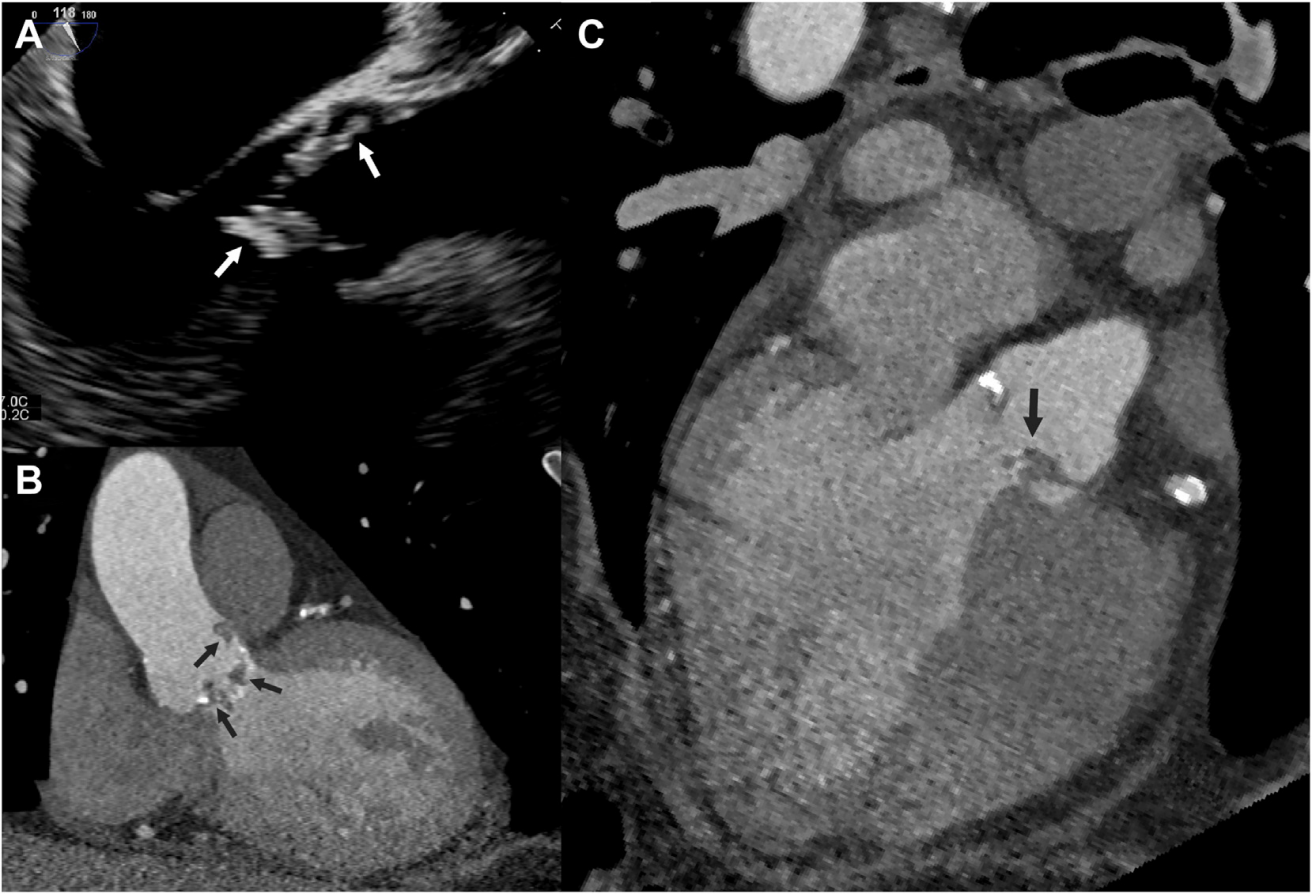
Patient with a native aortic valve endocarditis and vegetations. Vegetations (arrows) on the aortic valve and ascending aorta are seen on transesophageal echocardiography (A) and computed tomography (B and C).
